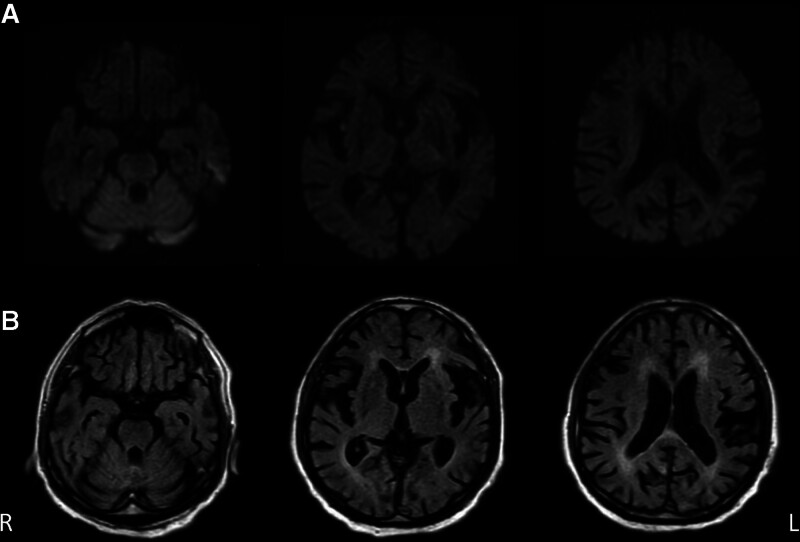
Brain magnetic resonance imaging (MRI) on admission. (A) Diffusion-weighted and (B) fluid-attenuated inversion recovery (FLAIR) images. Brain MRI showed mild atrophy of brain. FLAIR images indicated mild periventricular hyperintensity.
